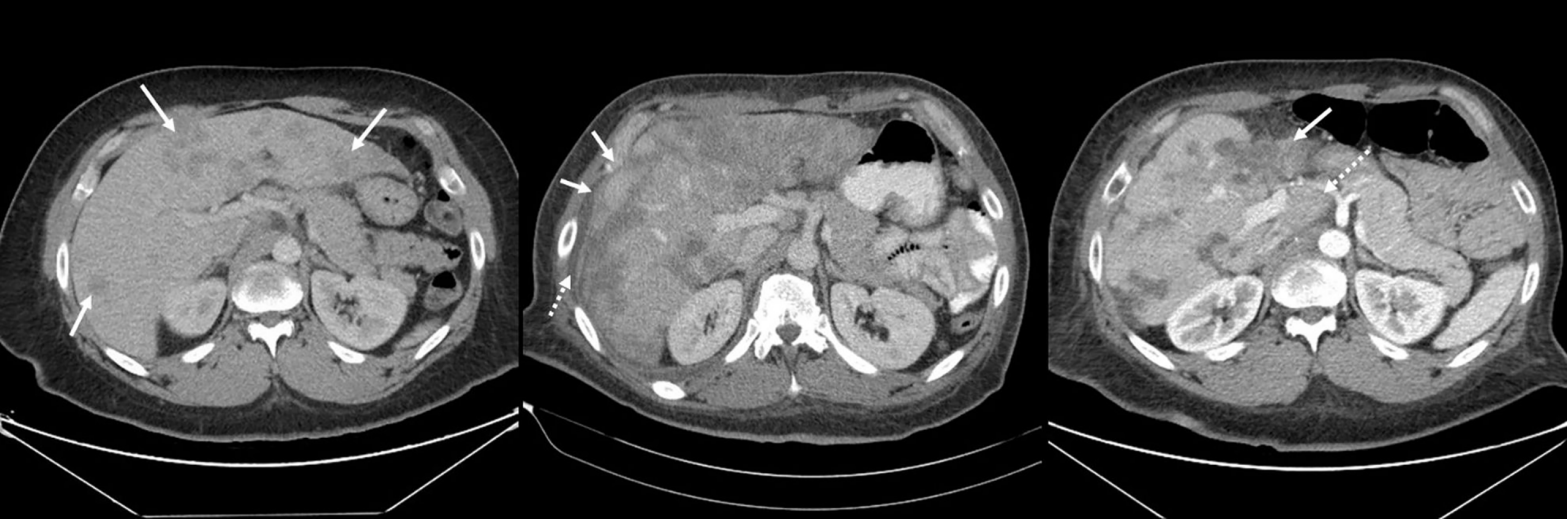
Serial contrast enhanced CT images of the abdomen in the axial plane showing evolution of pseudocirrhosis in a patient with breast cancer and liver metastases. (A) Baseline image shows multiple liver metastases (arrows); (B) At 6 months follow up, the lesions became larger and more confluent and the hepatic contour started to become nodular (solid arrows). A small volume of perihepatic ascites started to accumulate (dashed arrow). (C) At 13 months, there was significant atrophy of the left lobe (solid arrow) and hypertrophy of the caudate lobe (dashed arrow).
